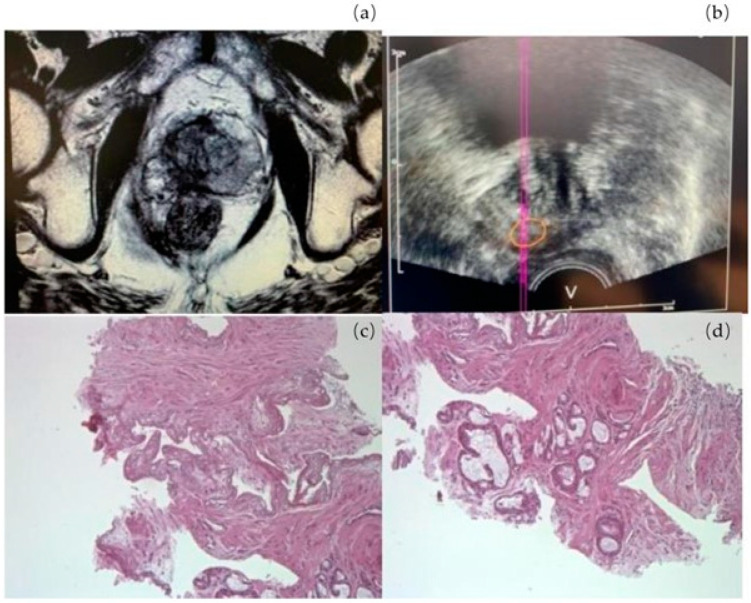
T2-weighted axial MRI images (PIRADS 4) (a); fusion biopsy (b); tumor area GS 6 (c); area of necrosis (d). The line and circle represent a machine function during biopsy.
